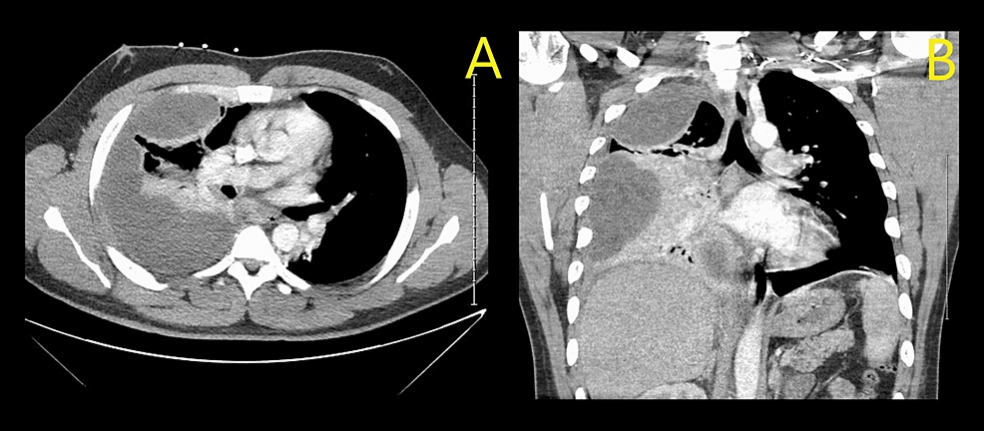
CT scans of the chest without contrast demonstrating loculated pleural effusion and atelectatic lung beneath it (A) transverse view and (B) coronal view.
